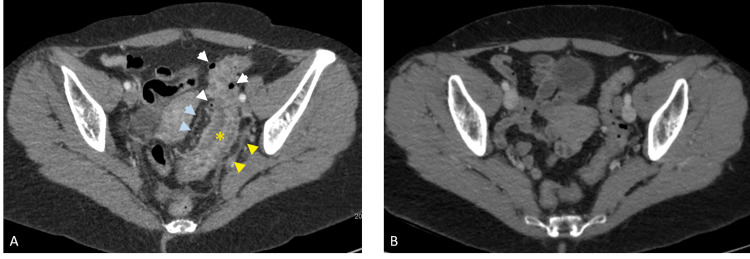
Abdominal CT at the time of patient’s presentation and after management (A) The axial CT image shows segmental sigmoid colon wall thickening (yellow asterisk), mild pericolonic inflammation (yellow arrowheads) sparing colonic diverticula (white arrowheads), and engorged vasa recta (blue arrowheads). (B) The axial contrast-enhanced CT image obtained after management shows resolution of sigmoid colon wall thickening and surrounding inflammation.
